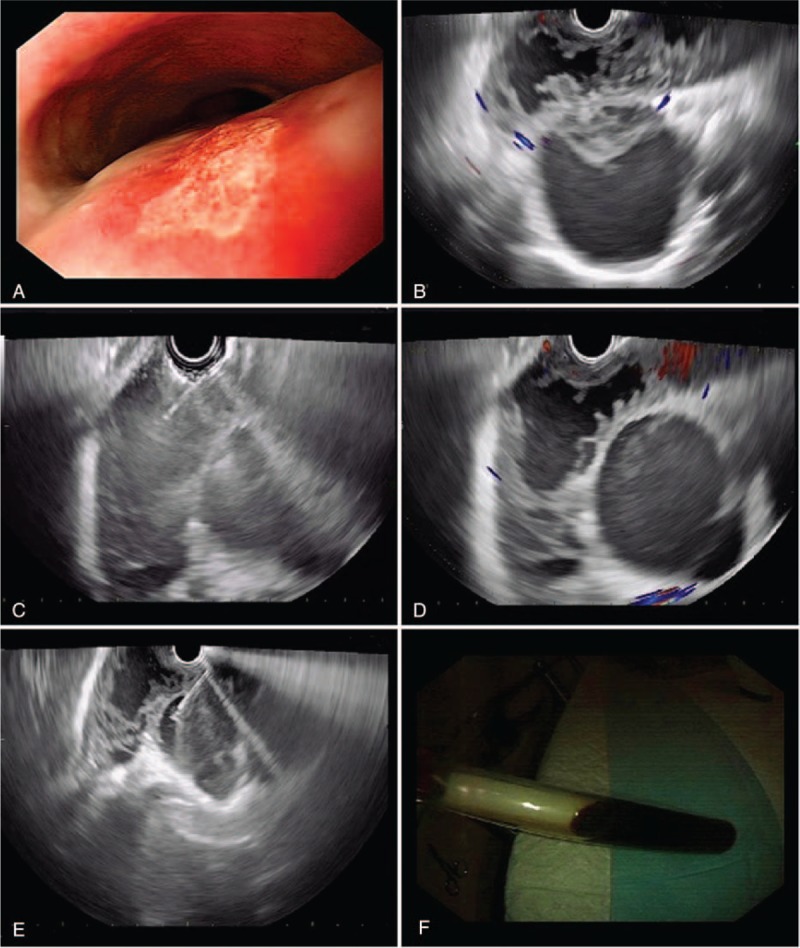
The characteristics of EUS appearance. (A) Upper endoscopy revealed a large ovoid bulging mass, 2.5 cm in diameter, with an erosion surface in the right anterior wall of the esophagus (arrowhead). (B–E) EUS evidenced that proper muscularis propria of the esophagus was damaged and tumor parenchyma was hypoechoic cystic-solid, concomitant with a heterogeneous center and tube-like structure lesion in mediastinum. (F) Concentrated coffee color fluid contents were aspirated by EUS-FNA. EUS = endoscopic ultrasonography, EUS-FNA = endoscopic ultrasonography guided-fine needle aspiration.
